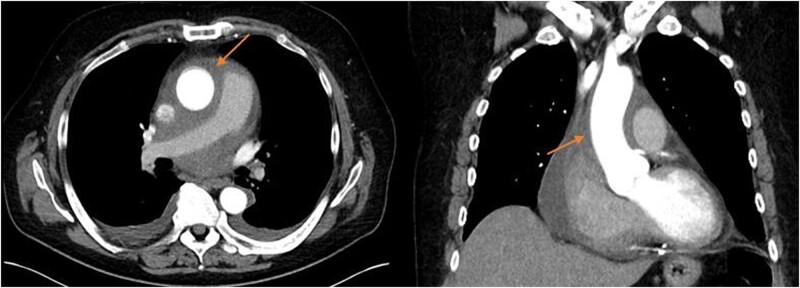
CT of the thorax showing the typical circumferential soft tissue sheathing of the thoracic aorta known as ‘coated aorta’.
